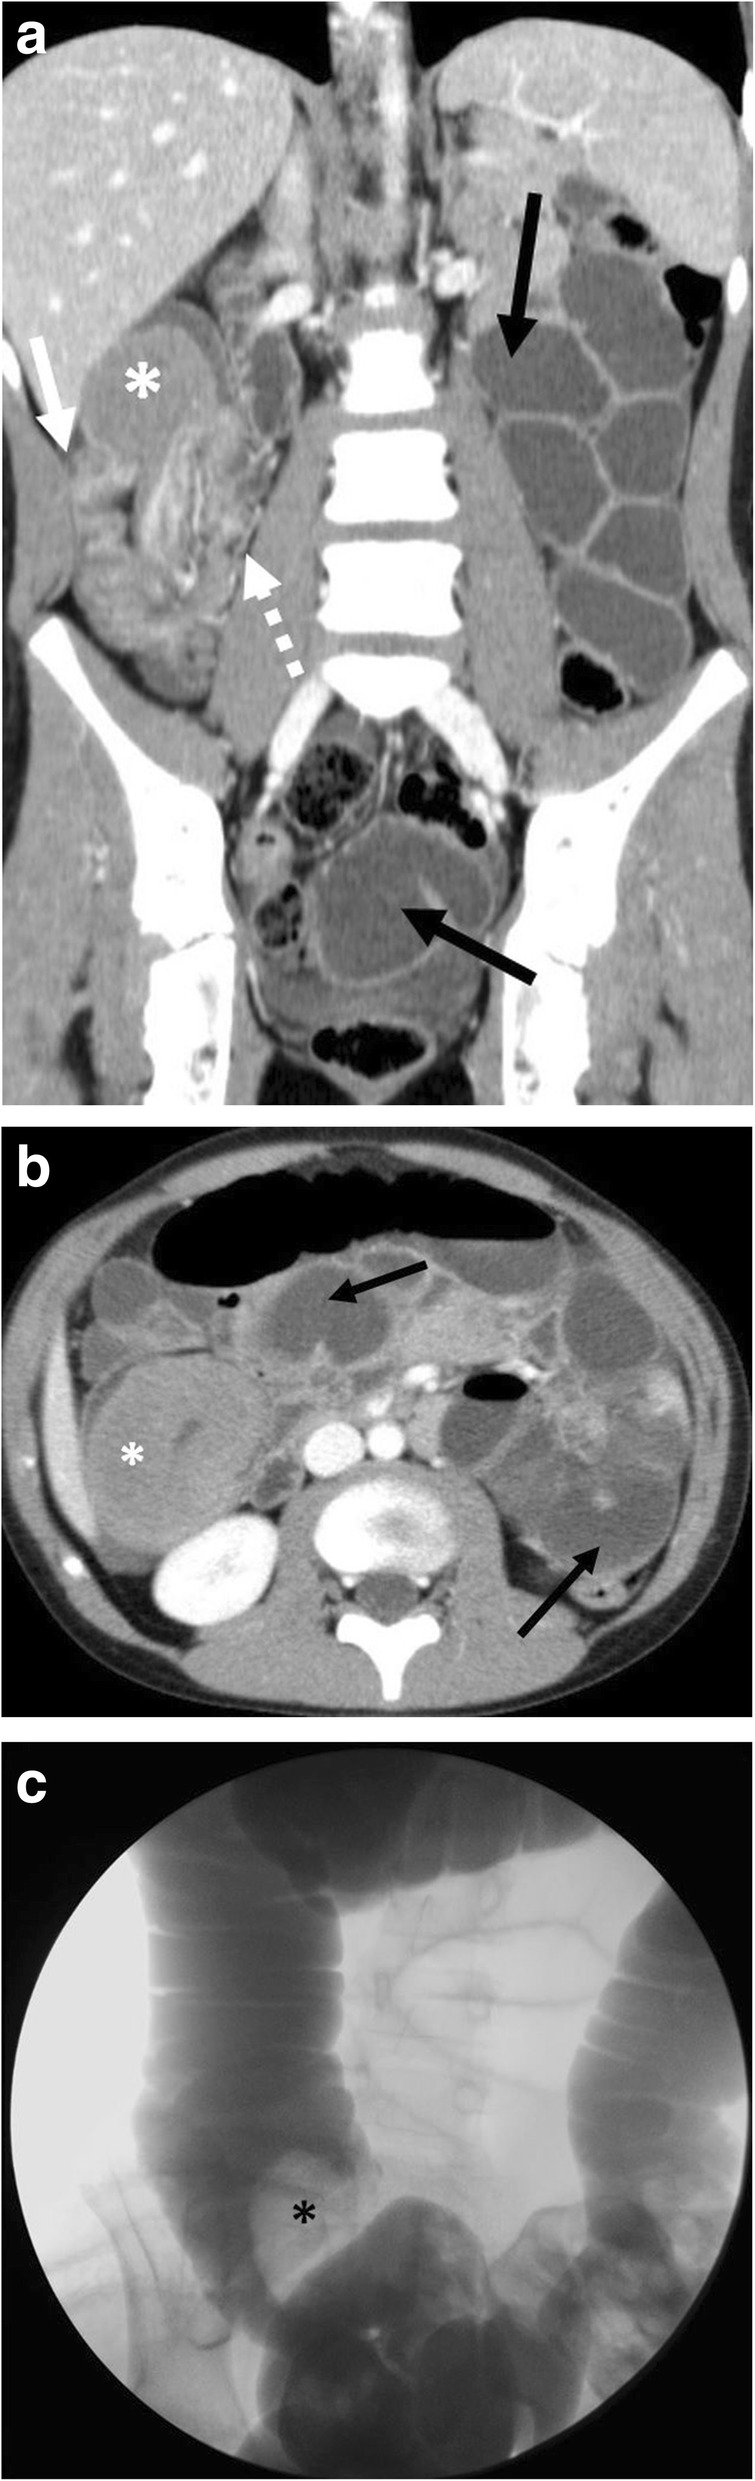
Bowel involvement with intussusception. A 10-year-old male presenting to the emergency department with abdominal pain. a Coronal and (b) axial contrast enhanced CT images demonstrate ileo-colic intussusception with intussusceptum (dashed arrow) and intussuscipiens (solid arrow) and dilated, fluid-filled small bowel loops (black arrows) from an associated small bowel obstruction. Lead point mass (asterisks) is also seen. c Subsequent fluoroscopic-guided contrast reduction enema demonstrating filling defect (asterisks) corresponding to the lead point mass was ultimately unsuccessful. The patient underwent surgical resection with pathology demonstrating Burkitt lymphoma
